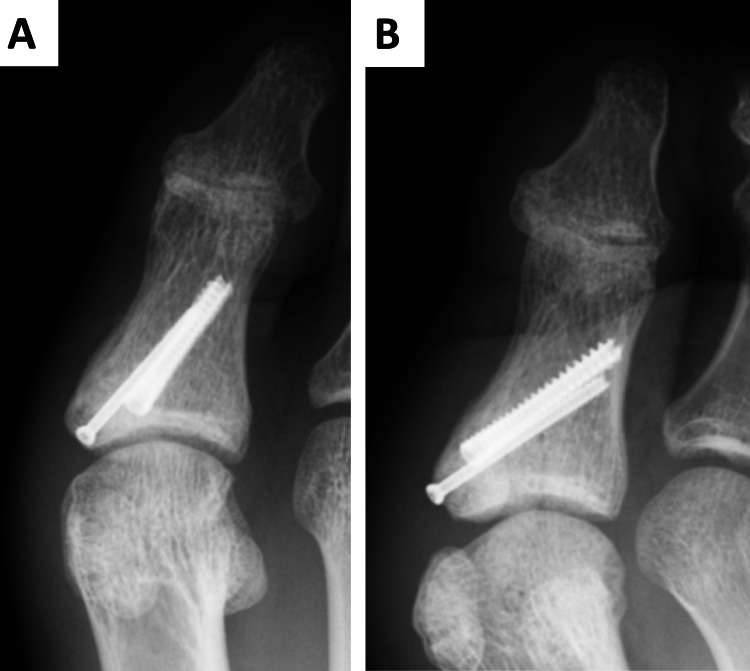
Radiography five months after the operation showed a solid union A: anteroposterior (AP) view; B: oblique view
